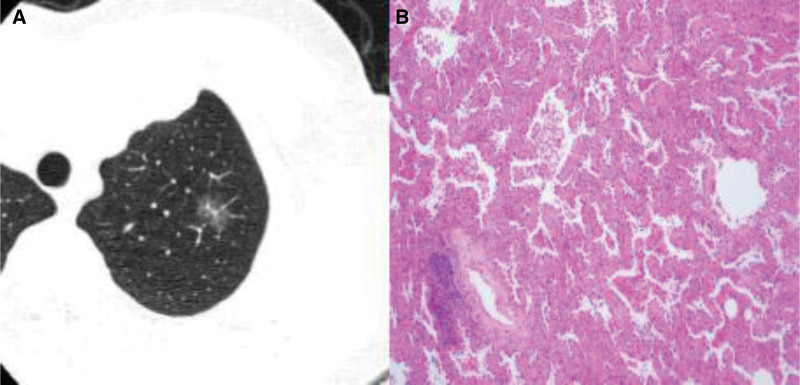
(A) The CT image and (B) the pathological image.
